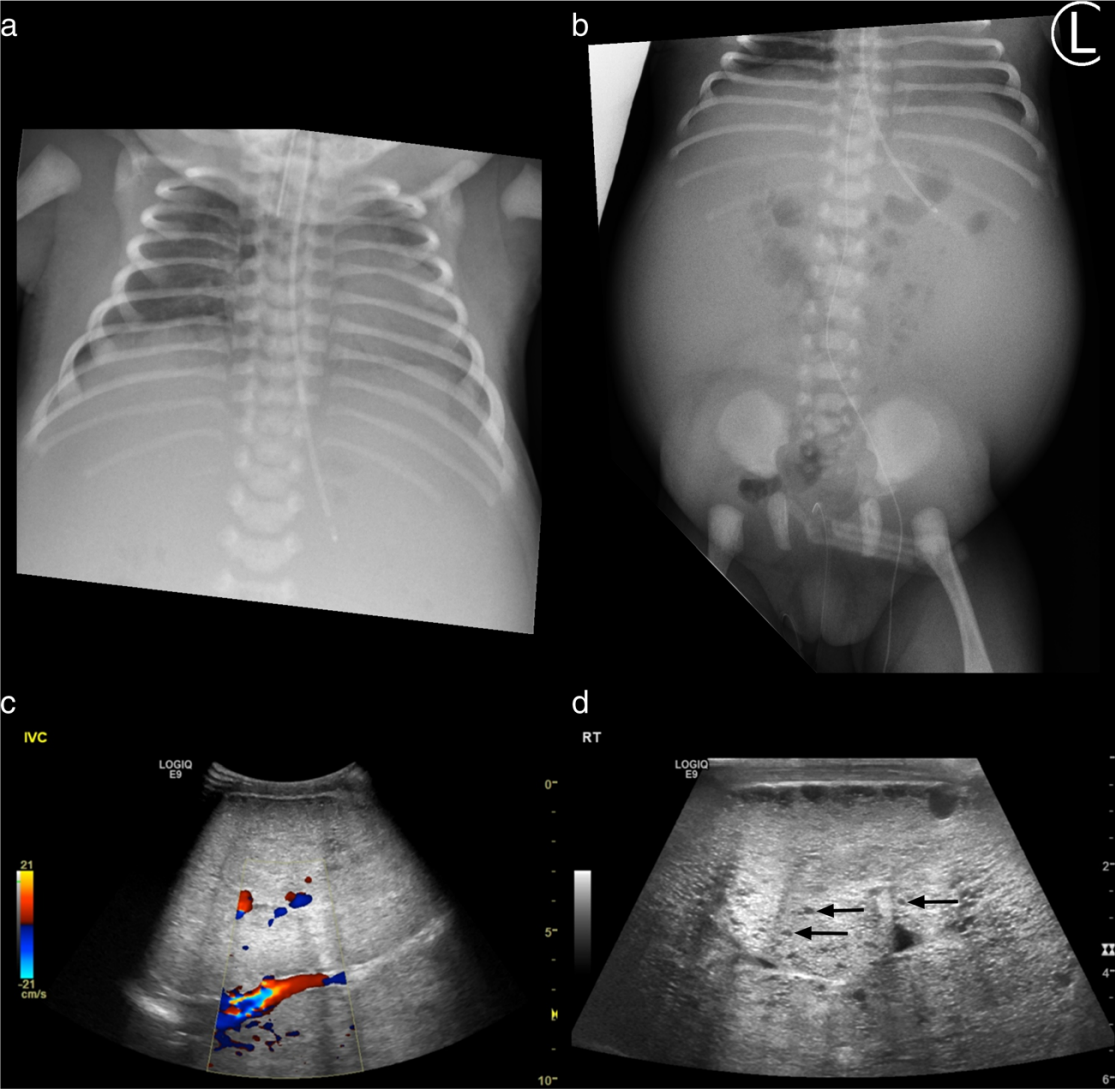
a Chest radiograph showing distended abdomen and small chest. b Abdominal radiograph showing distended abdomen with bilateral soft tissue masses and central bowel gas, also seen within a right inguinal hernia. c Abdominal ultrasound scan showing extremely enlarged and echobright kidneys with reduced corticomedullary differentiation compressing the inferior vena cava. The right kidney measured 10.2 cm long and the left 10.7 cm. The depth of both kidneys was 5.6 cm and transverse dimension 7.5 cm. At term, mean sonographic renal length was 4.48 ± 0.31 cm. d High-resolution view showing multiple small cysts, three of which are indicated by *arrows*
